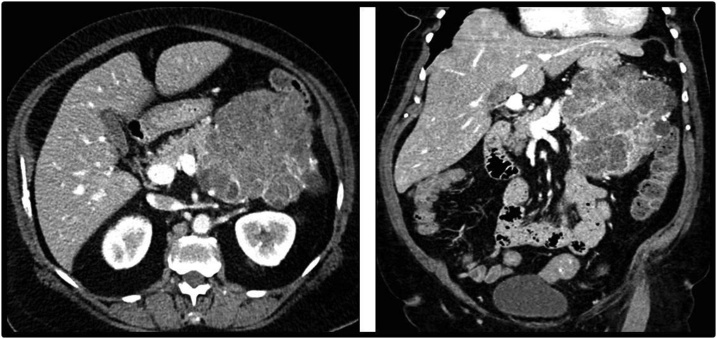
Computed tomography (CT) Imaging of Giant Serous Cystadenoma. Axial image, venous phase. Complex polycystic mass involving the entirety of the pancreatic body and tail measuring 12.4 x 11.0 cm in axial plane. Close proximity to surrounding visceral and vascular structures with concern for locally aggressive infiltration of this tumor. Coronal image, venous phase. Measuring 13.0 cm in craniocaudal dimension.
